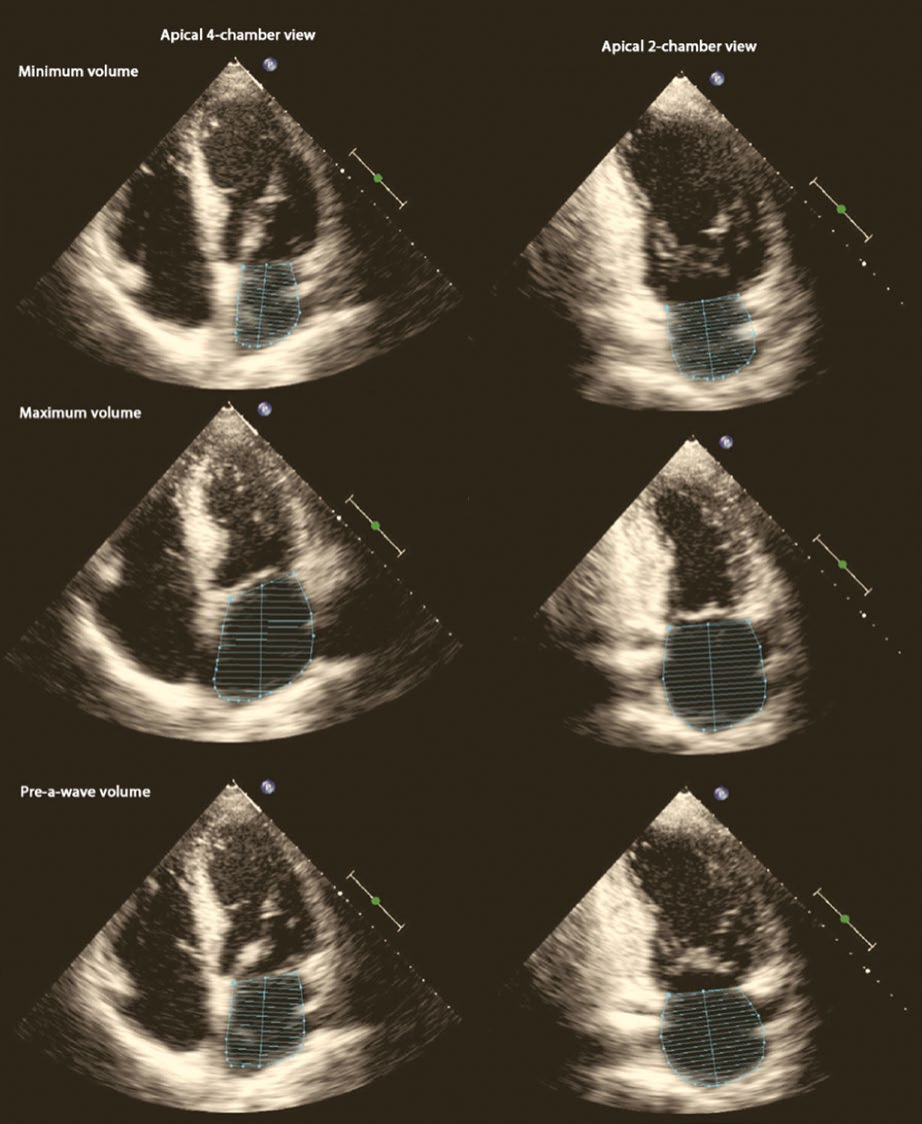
Example of the volumetric measurements using the method‐of‐disk summation technique in dedicated apical four‐ and two‐chamber views. From top to bottom: the left atrial minimum, maximum, and pre‐a‐wave volume
